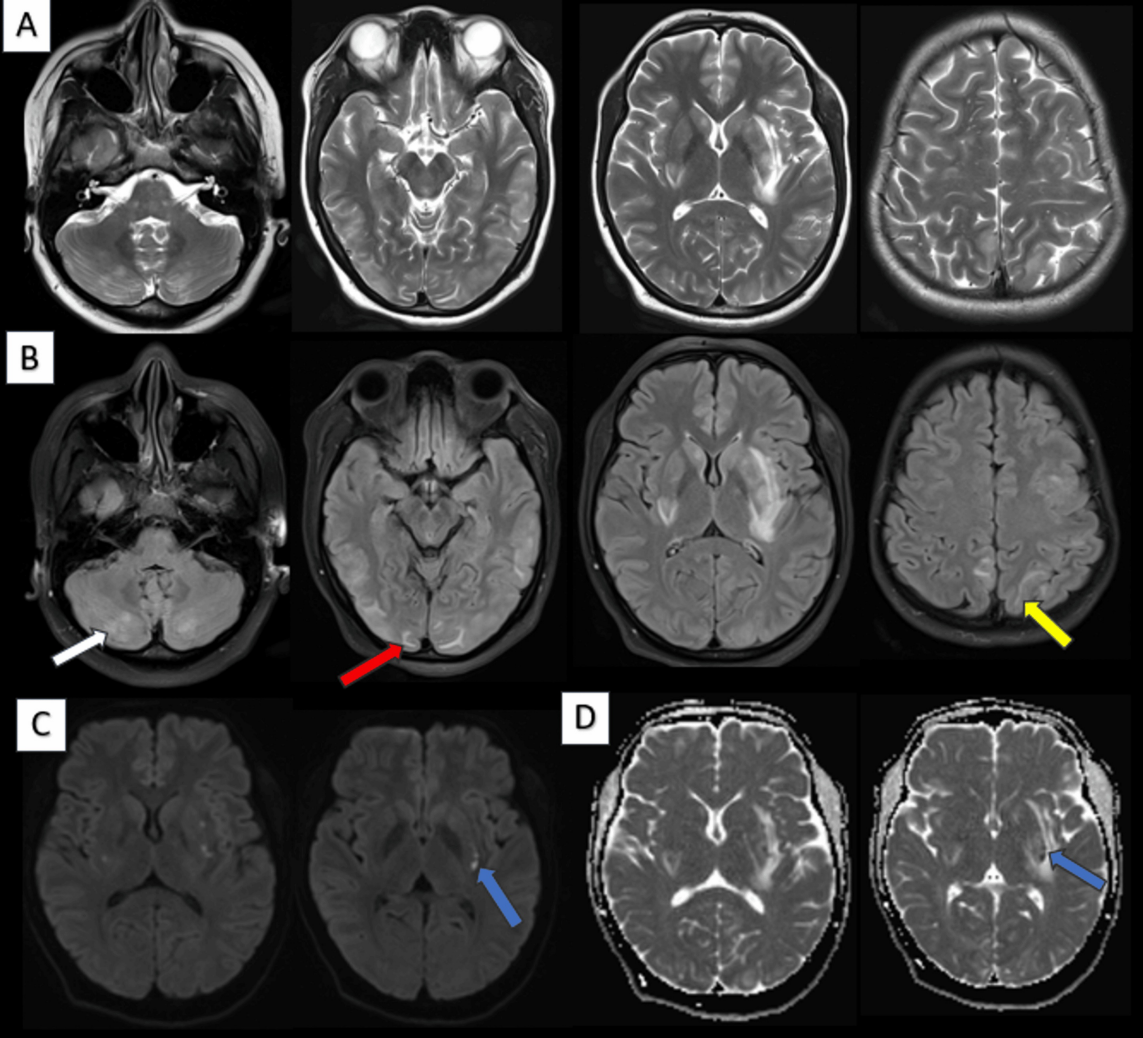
Axial T2W (A), FLAIR (B), DWI (C), and ADC (D) brain MRI. A 24-year-old female presented with a headache and two episodes of seizures on postpartum day seven. Axial brain MRI (A, B) showed T2W/FLAIR hyperintensities in both cerebellar hemispheres (white arrow), bilateral basal ganglia, subcortical white matter of the bilateral temporo-occipital (red arrow), parietal (yellow arrow), and left frontal lobes. Small foci of diffusion restriction with ADC reversal were observed in the bilateral basal ganglia (blue arrow, C and D). T2W: T2-weighted; FLAIR: fluid-attenuated inversion recovery; DWI: diffusion-weighted imaging; ADC: apparent diffusion coefficient.
